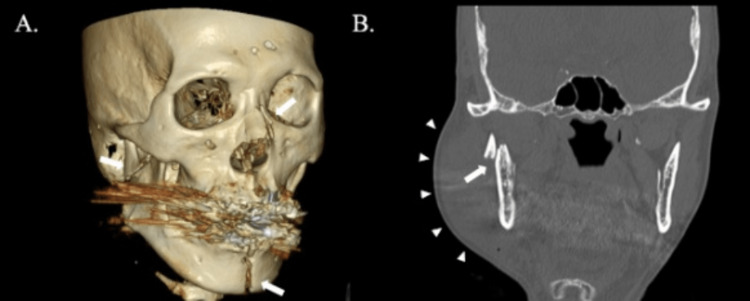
Facial computerized tomography (CT) on admission (A) Facial three-dimensional (3D) reformatted CT images on admission. Fractures of the left mandible body, right mandible head and neck, and nasal bone are observed (white arrows). (B) Coronal section of facial CT image on admission. Right mandible head fracture (white arrow) and soft tissue swelling are observed (white arrowheads)
